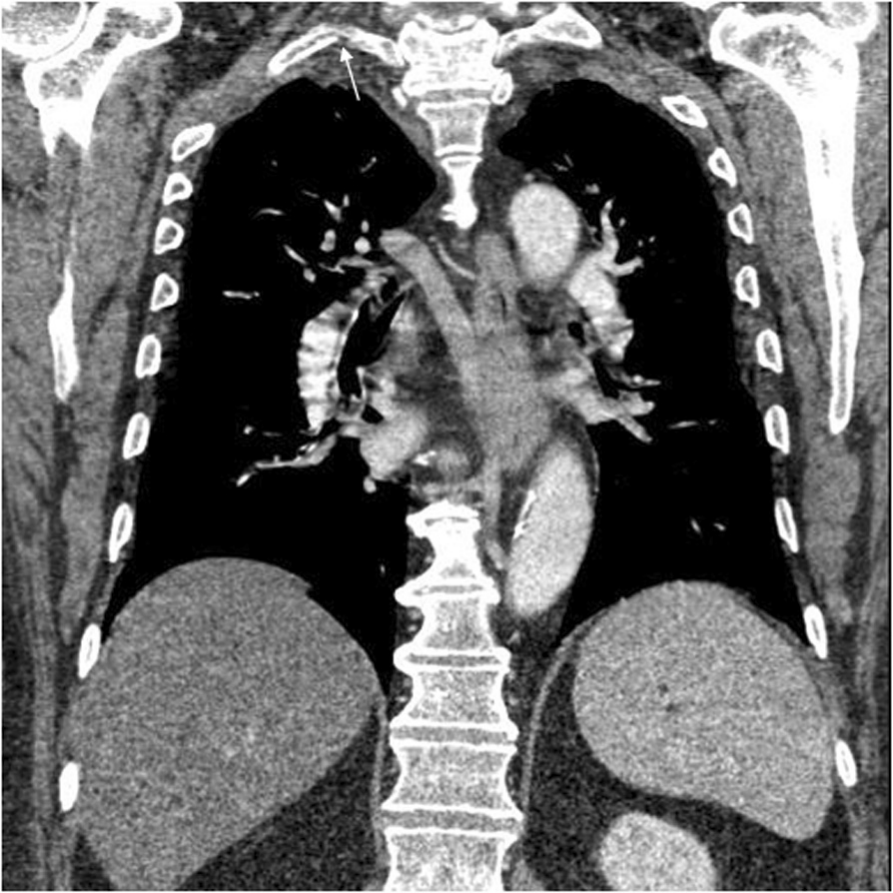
CT of the chest with contrast. This coronal view reveals a displaced fracture fragment of the proximal right first rib (white arrow).
